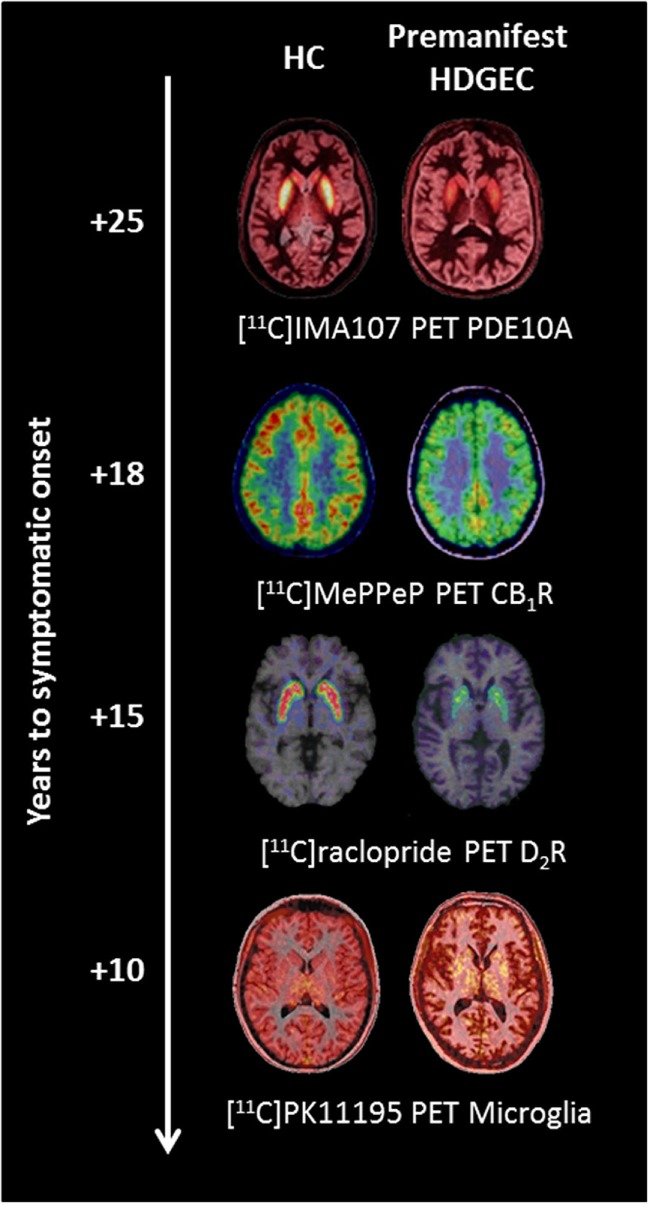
Changes in molecular positron emission tomography (PET) biomarkers in premanifest Huntington’s disease expansion gene carriers (HDGECs) compared to healthy controls (HC) as the disease progresses toward symptomatic onset. Axial [^11^C]IMA107, [^11^C]MePPeP, [^11^C]PK11195, and [^11^C]raclopride PET images coregistered and fused to corresponding T1-weighted MRI to show changes in premanifest HDGECs compared to HC. Earliest loss of striatal phosphodiesterase 10A (PDE10A) is detectable on average 25 years before symptomatic onset, followed by loss of cortical cannabinoid type 1 receptor (CB_1_R), increased microglia activation, and loss of striatal dopamine type 2 receptor (D_2_R) binding. Images produced from our data.
